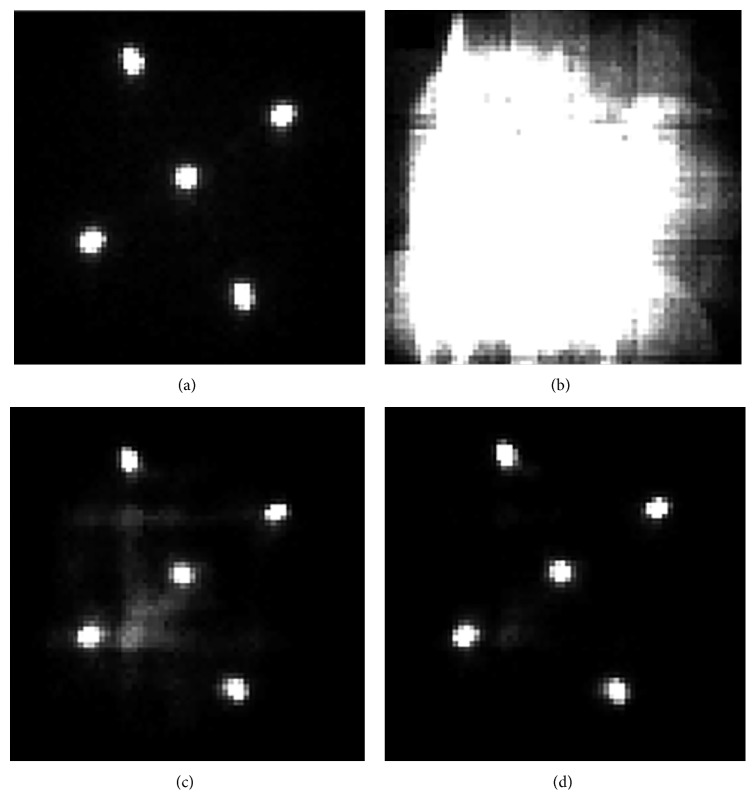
SPECT projections of a ^57^Co point source using a 5-pinhole tungsten collimator. (a) 30–300 keV energy window and no X-rays present, (b) 30–300 keV energy window and X-ray source on 80 kVp, (c) 110–134 keV energy window and X-ray source on 80 kVp, and (d) 110–134 keV energy window and X-ray source on 70 kVp.
